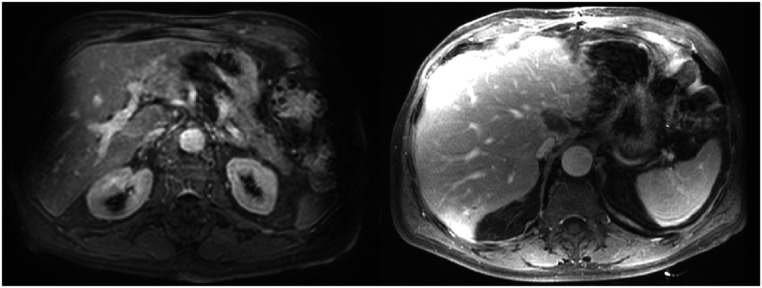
Example of gradient effects in MRI that may increase DIR uncertainties. Figure from (Vásquez Osorio *et al*
